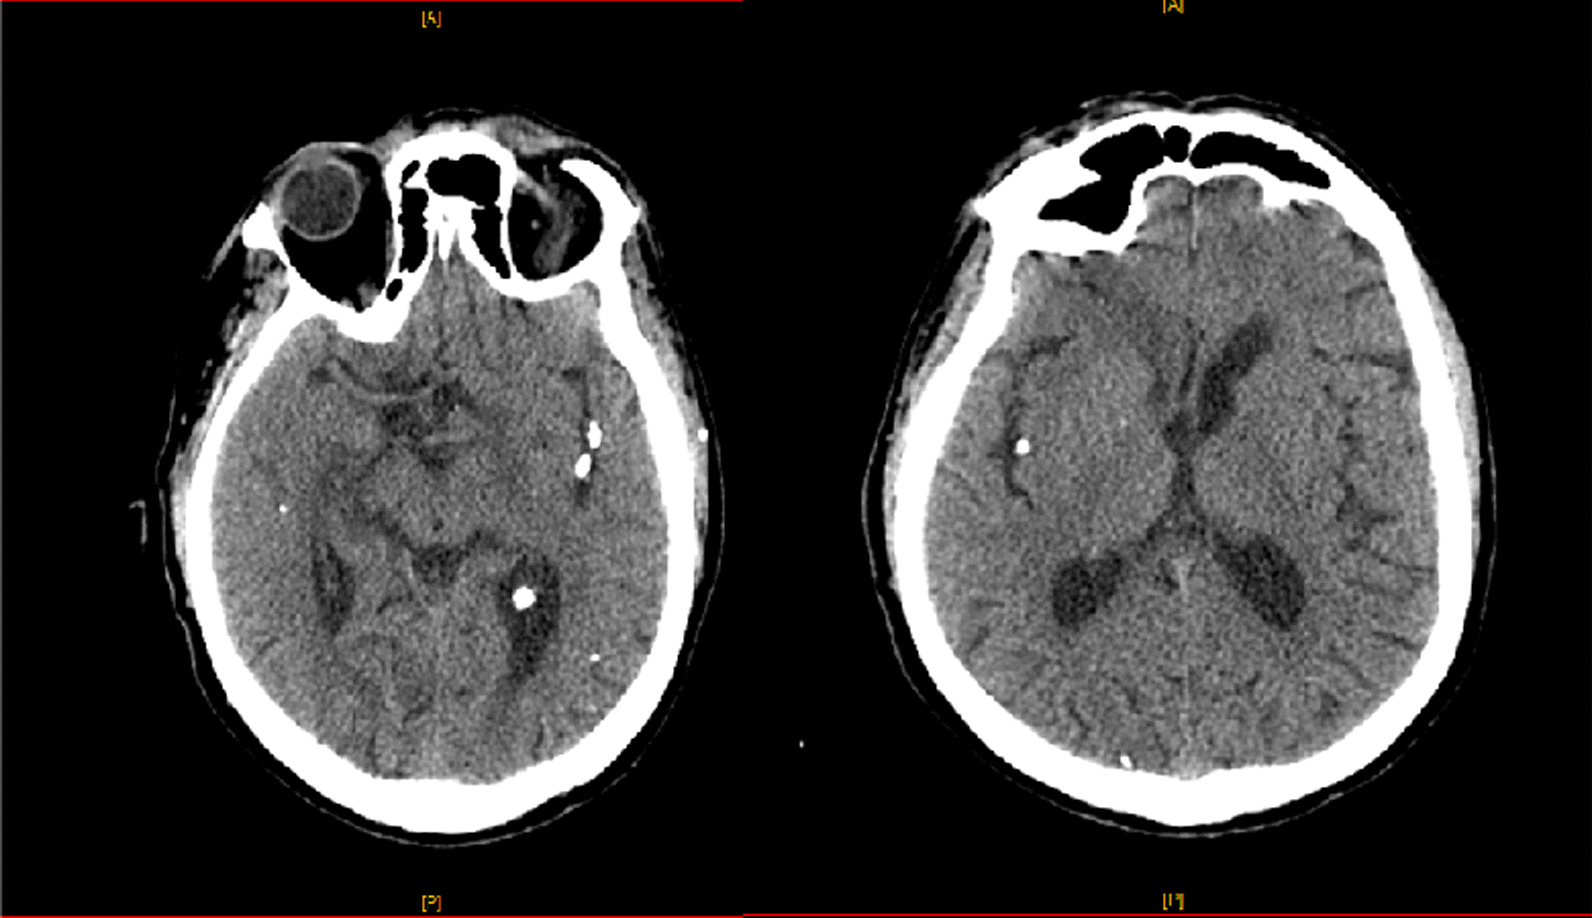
Unenhanced cerebral computed tomography with axial reconstructions showing calcified emboli in both middle cerebral arteries. These arteries were permeable
